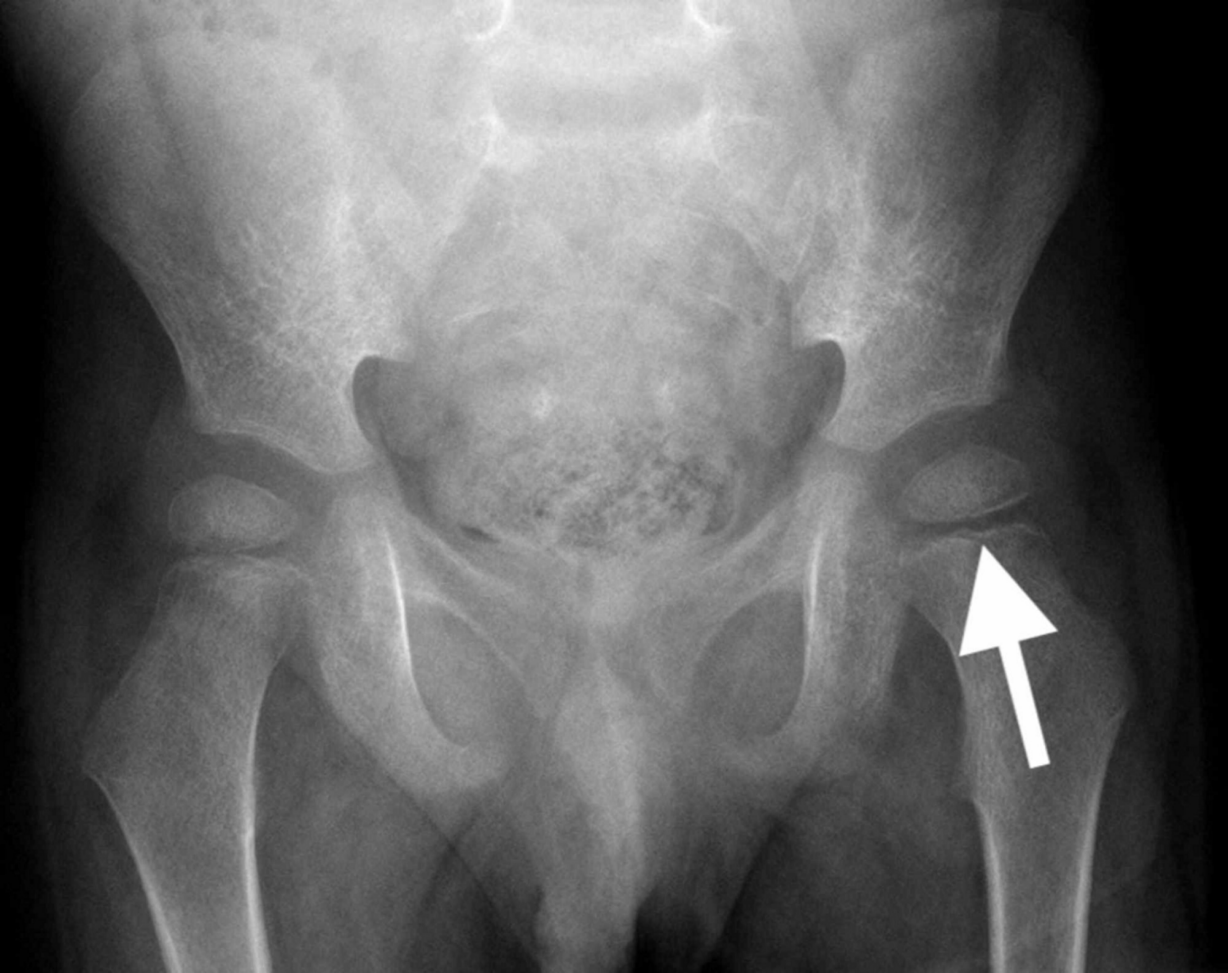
X-ray of the pelvis (anteroposterior view) from third presentation to hospital (arrow pointing to a subcortical osteoporotic ring within the femoral capital epiphysis which is more subtle in this view)
